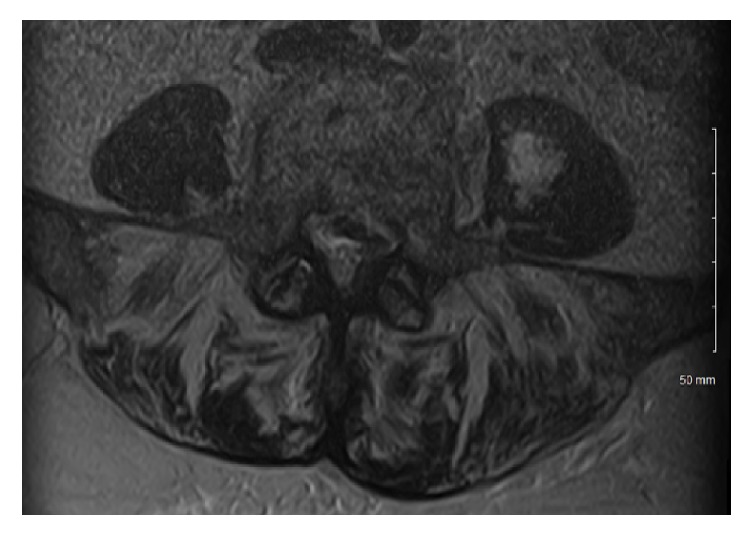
MRI of the lumbar spine on hospital day 3. This MRI shows a fluid collection within the left psoas muscle. The collection was aspirated and revealed purulent brown material, consistent with an abscess.
